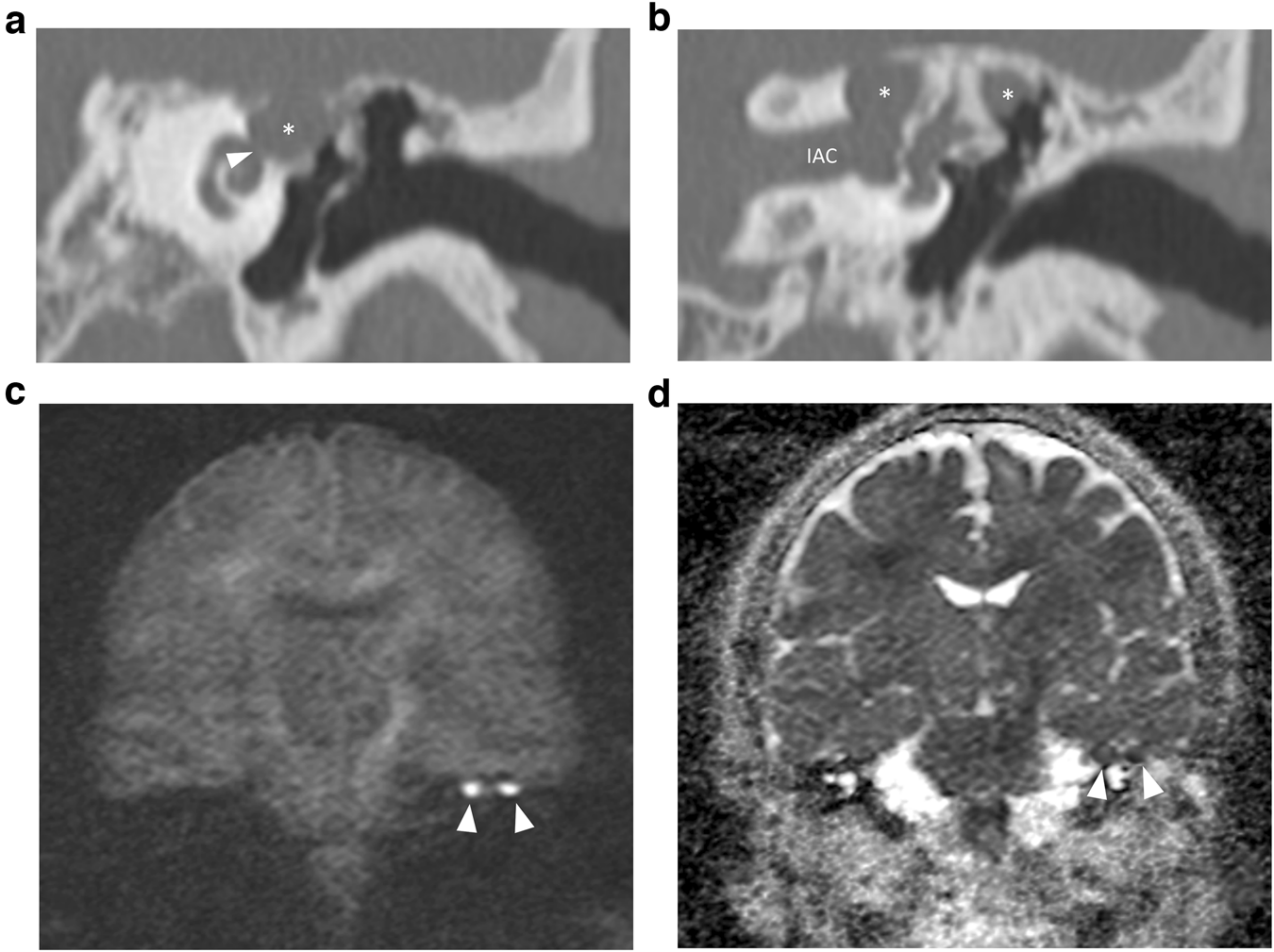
Supralabyrinthine (Class I) CC of the left temporal bone in a 57-year-old female patient presenting with peripheral facial paresis and mixed hearing loss on the left side. Coronal reformatted CT images of the left temporal bone show a CC in the supralabyrinthine region in close relation to the geniculate ganglion (asterisk in a) and dehiscence of the middle cochlear turn (arrowhead in a). The CC extends further backward, medial, and lateral to the superior semicircular canal (asterisks in b) with a large dehiscence of the IAC. Non-EP DWI and ADC map (c, d) at the same level as (b) show true restricted diffusion consistent with the diagnosis of a CC (arrowheads in c, d)
